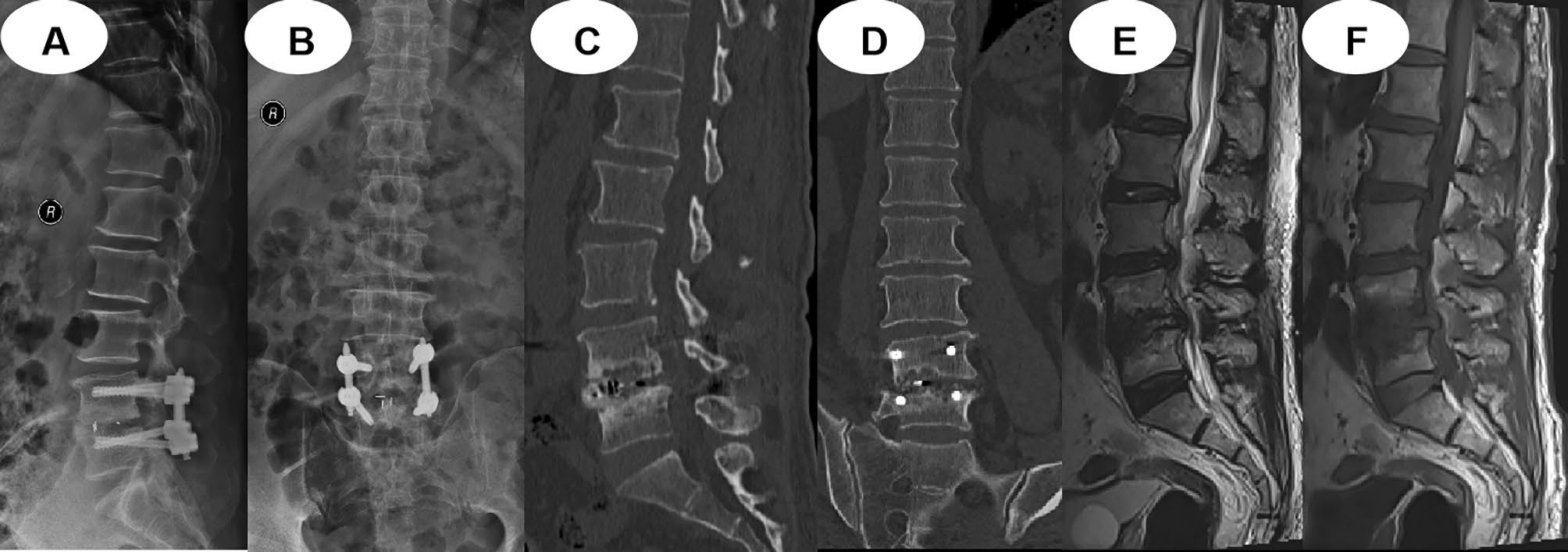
A 65-year-old man had intervertebral infection after Endo-TLIF. A-P and lateral plain film of the lumbar vertebra showed narrowing of the intervertebral space (A, B); sagittal and coronal CT showed that the vertebrae were rough, blurry, broken and sclerotic (C, D); sagittal T1- and T2-weighted MRI showed intervertebral infection (E, F) at 6 months after surgery.
